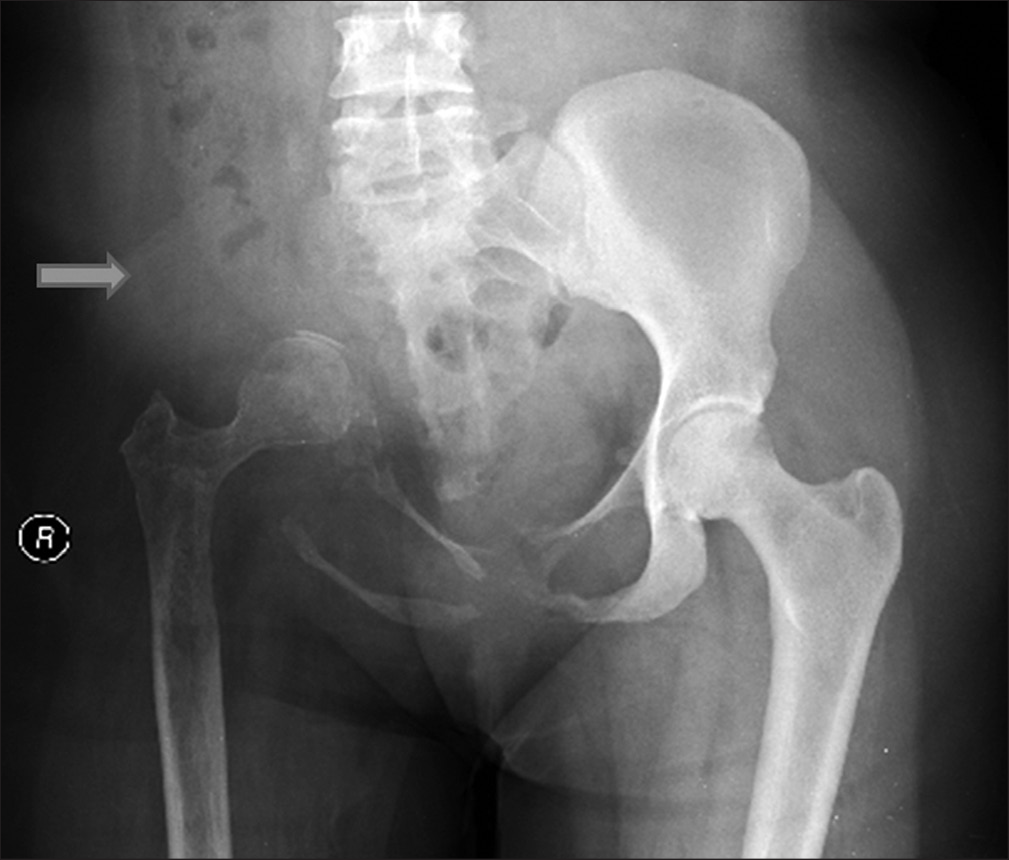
Pelvic X-ray shows absent bone tissue in the right iliac bone, right sacroiliac joint, and part of the right ischial pubic rami (arrow).
